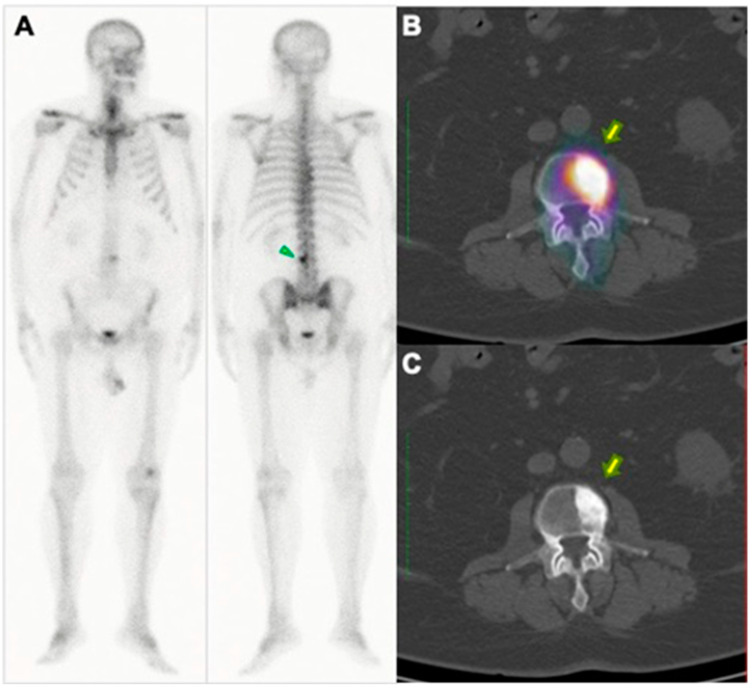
Example of conventional bone scintigraphy and SPECT/CT using the radiopharmaceutical ^99m^Tc-MDP of a 62-year-old prostate cancer patient (Gleason, 3 + 4; PSA equals 11.3 ng/dL) undergoing the evaluation for primary staging. (A) Whole-body conventional images demonstrate a focal area of abnormal uptake (arrow) in the 3rd lumbar spine. The abnormal uptake was equivocal for metastases. Therefore, the patient underwent SPECT/CT images. The axial images in the fused SPECT/CT (B) and corresponding CT (C) demonstrate increased activity in areas consistent with osteoblastic bone metastasis.
